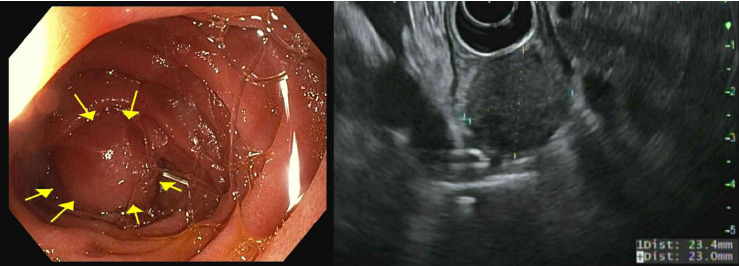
Endoscopic and ultrasound views of the submucosal duodenal mass.
